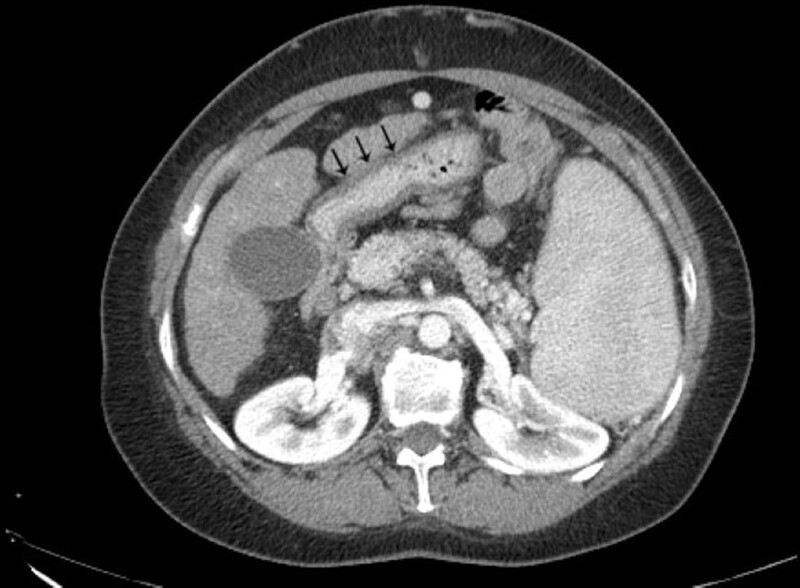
Duodenal wall thickening (black arrows) due to ischemia on contrast-enhanced CT scan. CT = computed tomography.
